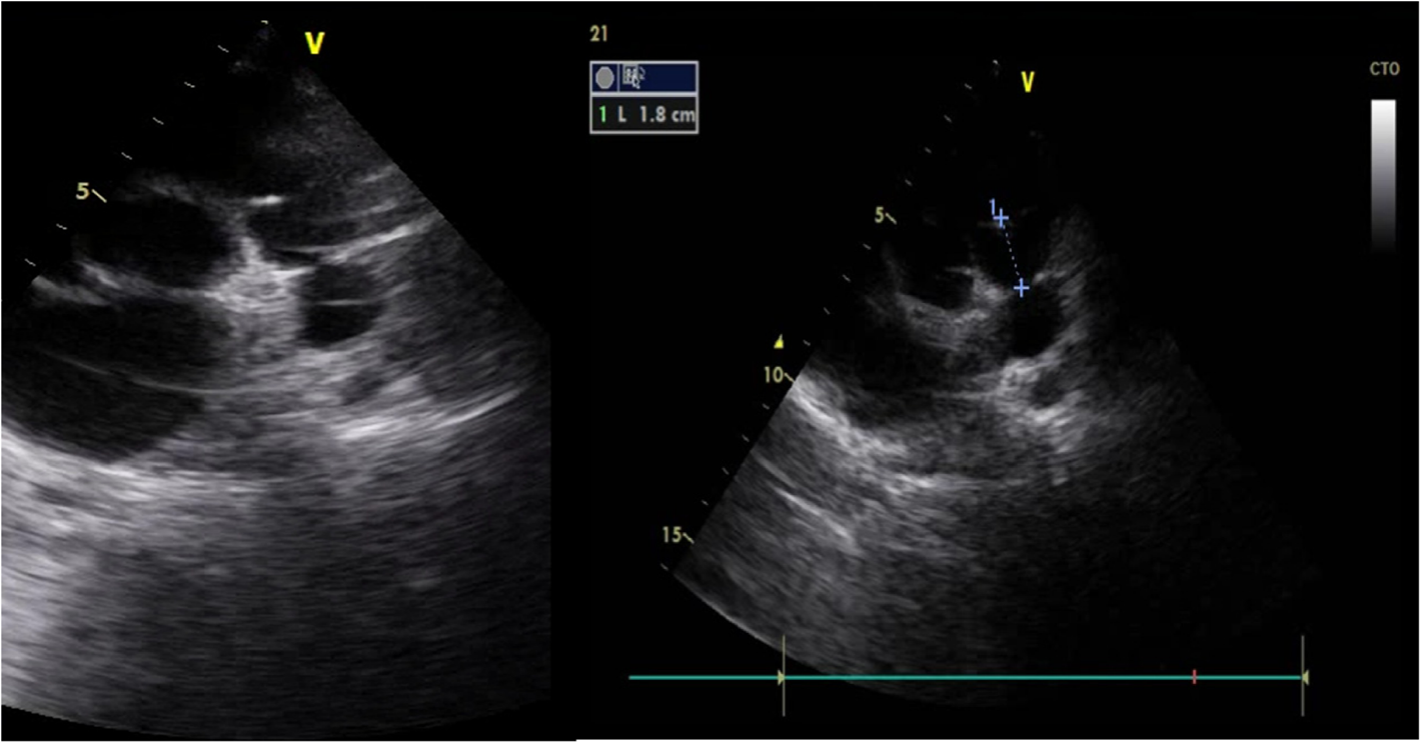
Parasternal short-axis view of a transthoracic echocardiogram showing subpulmonic membrane standing 18mm below pulmonic valve leaflets
